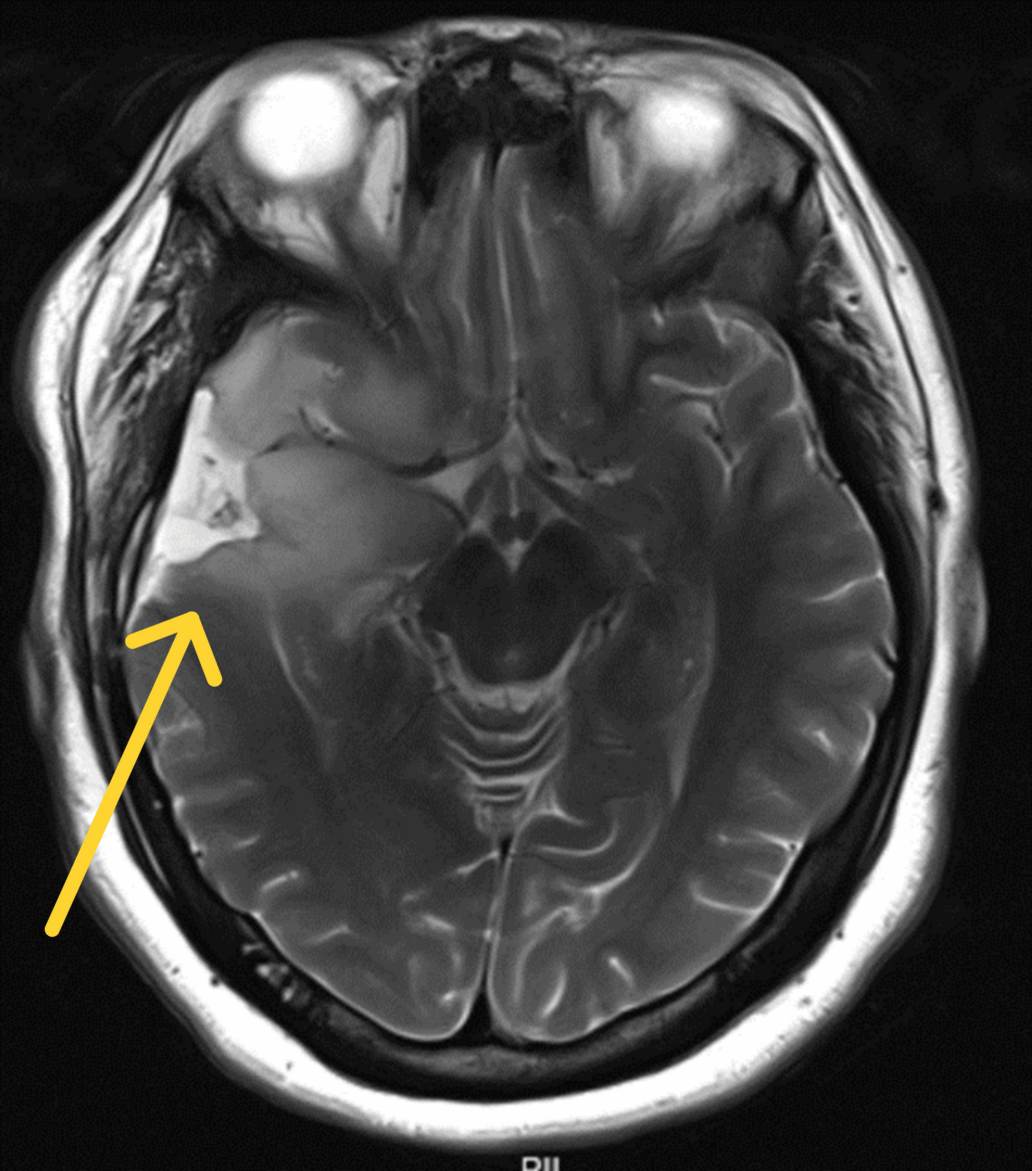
Follow-up MRI approximately 12 months postoperatively (T2-weighted axial cut) showing tumor recurrence in the right temporal lobe with associated edema.
